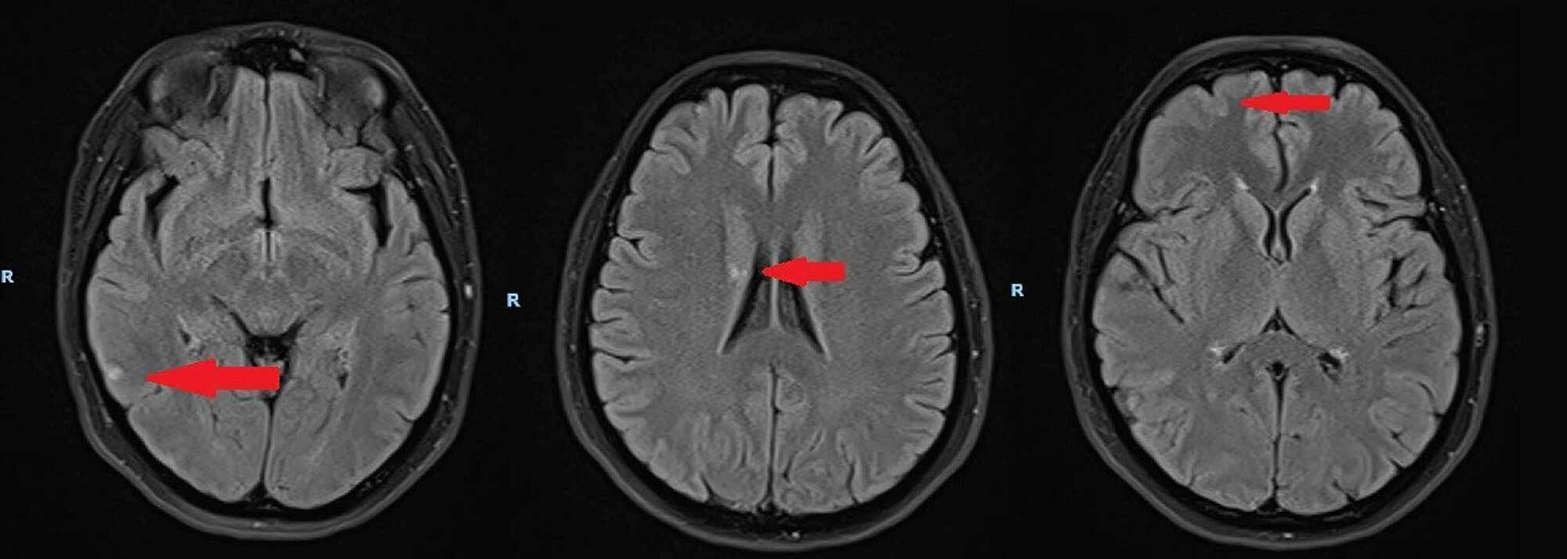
MRI Brain demonstrating areas of acute non-haemorrhagic infarcts
